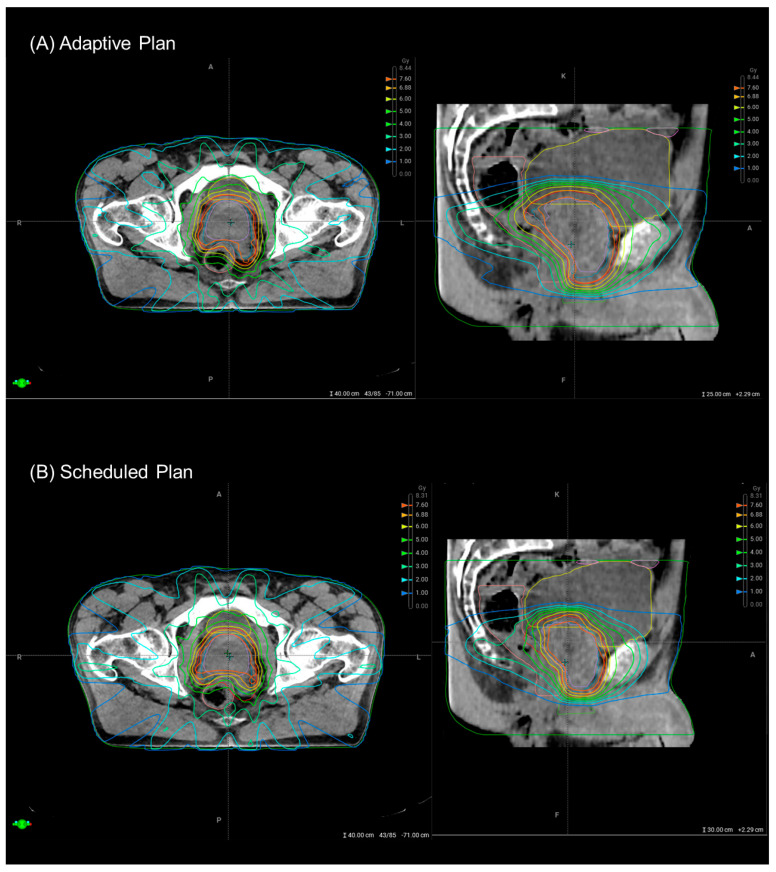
Comparison between an exemplary adaptive plan (A), based on the adaptation of structures in the current cone beam computed tomography (CBCT), and the corresponding scheduled plan (B), in which the initial dose distribution is superimposed onto the anatomy of the current CBCT. The adapted plan enables improved coverage of the planning target volume (PTV1, red), which includes the prostate and proximal seminal vesicles, particularly in posterior regions adjacent to the rectum (pink). At the same time, doses to the bladder (yellow) are reduced. The displayed isodose lines correspond to 95% of the prescribed dose for PTV1 (7.25 Gy) and PTV2 (boost to 8.00 Gy, light red structure comprising the prostate only).
